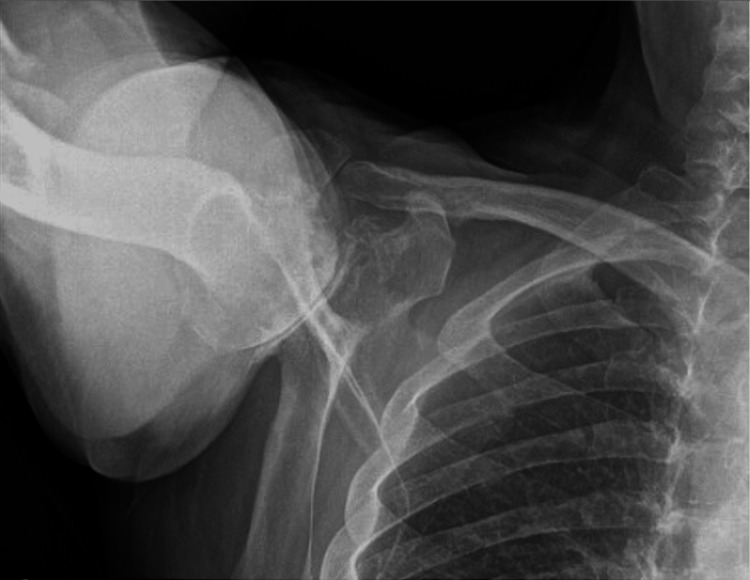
Preoperative X-rays of the right shoulder The image depicts an attempted axillary view of the right shoulder
